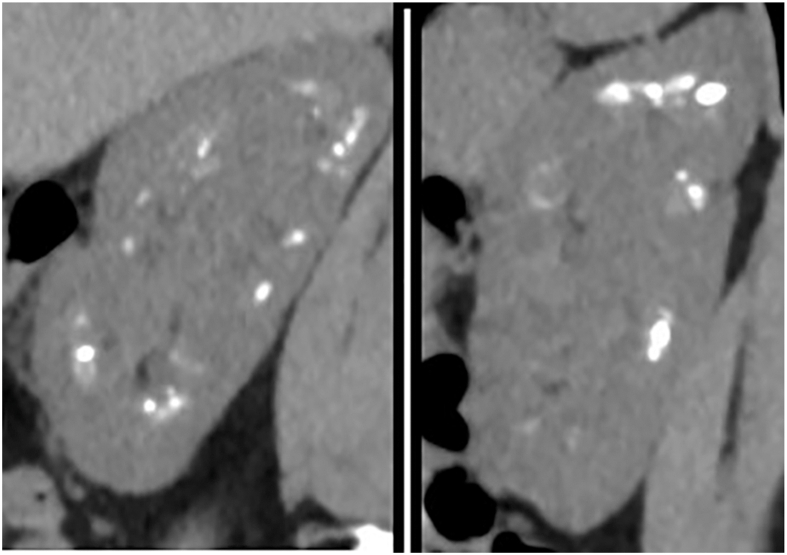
Non-contrast sagittal abdominal computed tomography images for Patient 1 revealed nephrocalcinosis.
